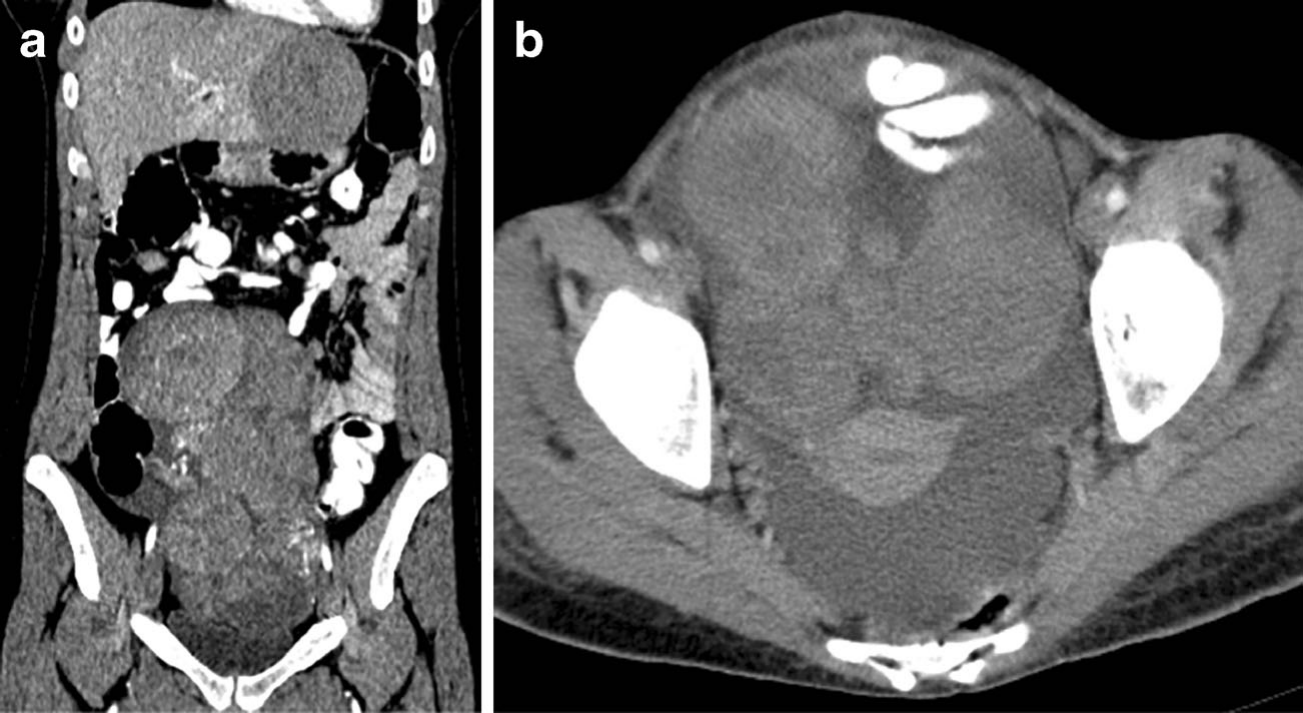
a–b Metastatic, bilateral ovarian carcinoid, from a primary jejunal tumour in a 28-year-old female complaining of pelvic pain and dyspareunia. Coronal (a) and axial (b) enhanced CT images. Multinodular, heterogeneous and bilateral ovarian tumour, with moderate enhancement after contrast administration. Ascites are found in the pelvis and parieto-colic gutters. Multiple liver metastases were also seen (not shown)
